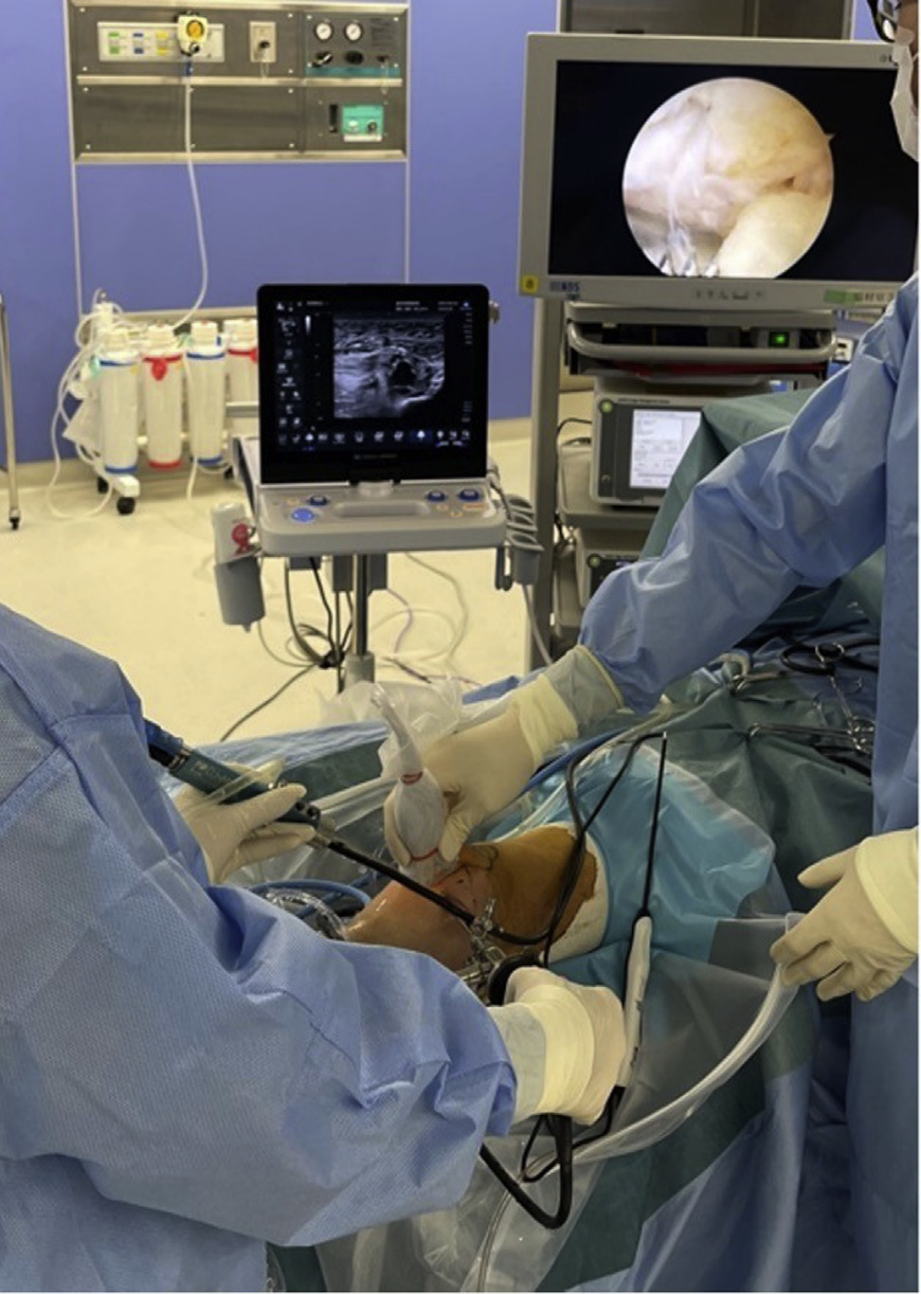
A situation in which the ossicle is being searched for using ultrasound. Intraoperative ultrasound imaging is performed by the surgical assistant. The surgeon identifies the ossicle by using an arthroscopic probe while looking at the ultrasonic monitor.
